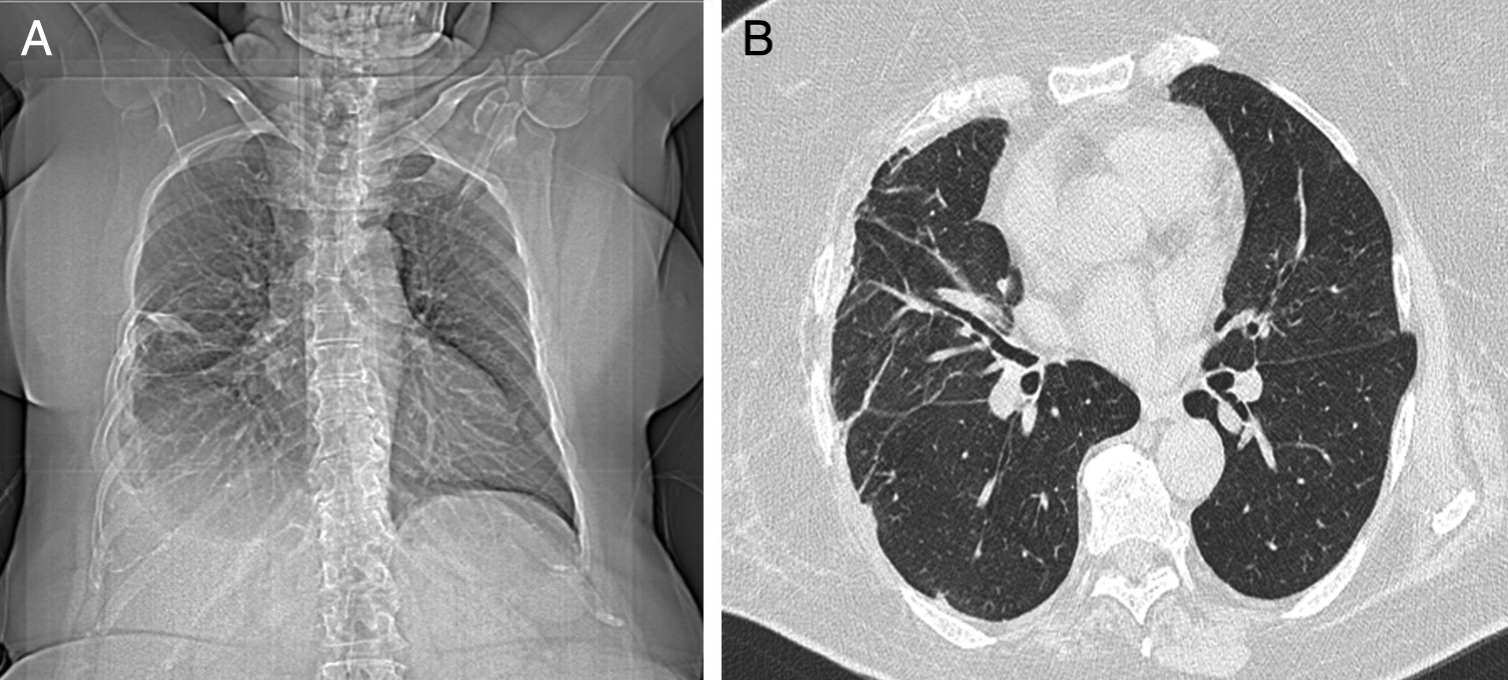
Chest computed tomography (CT) showing the radiographic resolution after surgical reduction (A and B).
